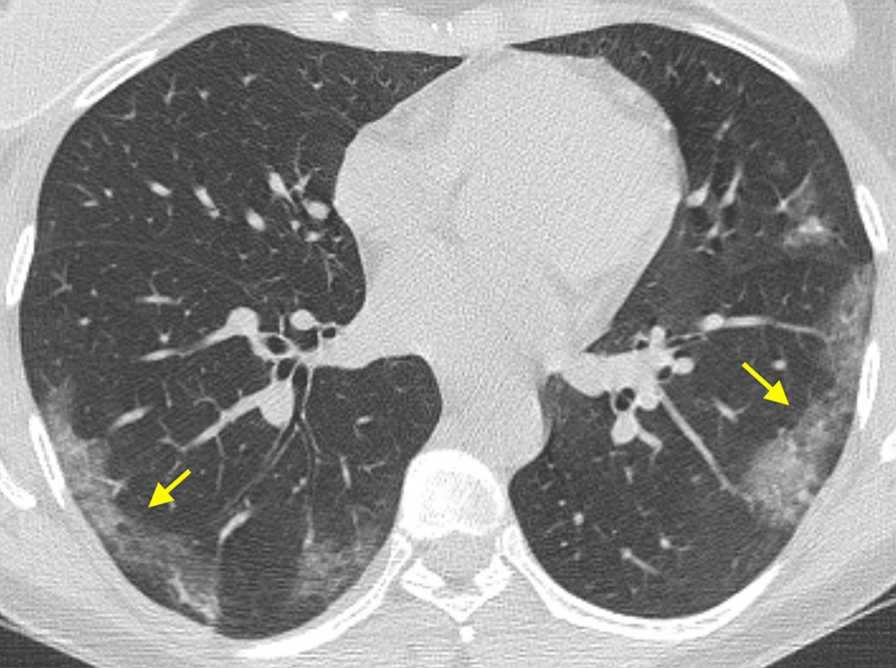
Typical chest CT imaging appearance of COVID-19 pneumonia. High-resolution CT image of a female COVID-19 patient presenting fever with cough for 5 days shows bilateral, multifocal, and peripheral areas of pure ground-glass opacity (GGO) (arrows)
